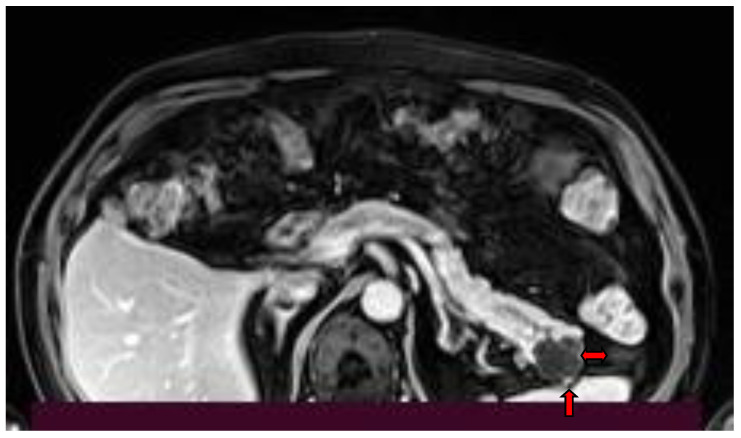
IPMN in the pancreatic tail, presenting as a cystic lesion on contrast-enhanced T1-w MR image. Enhancing mural nodules are obvious (arrows).
